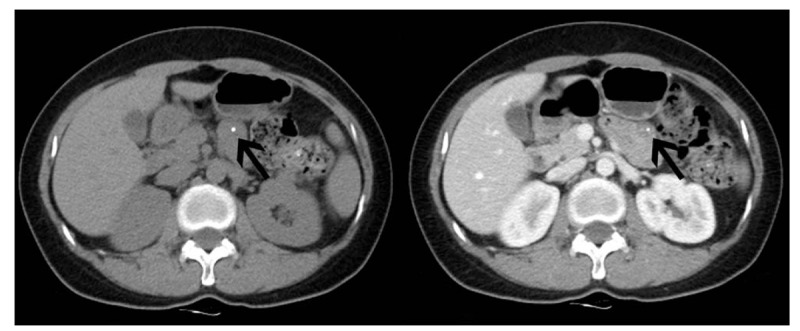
CT demonstrated a space-occupying lesion in proximal jejunum with calcium deposition, which had exhibited enhancement after contrast injection. CT = computed tomography.
